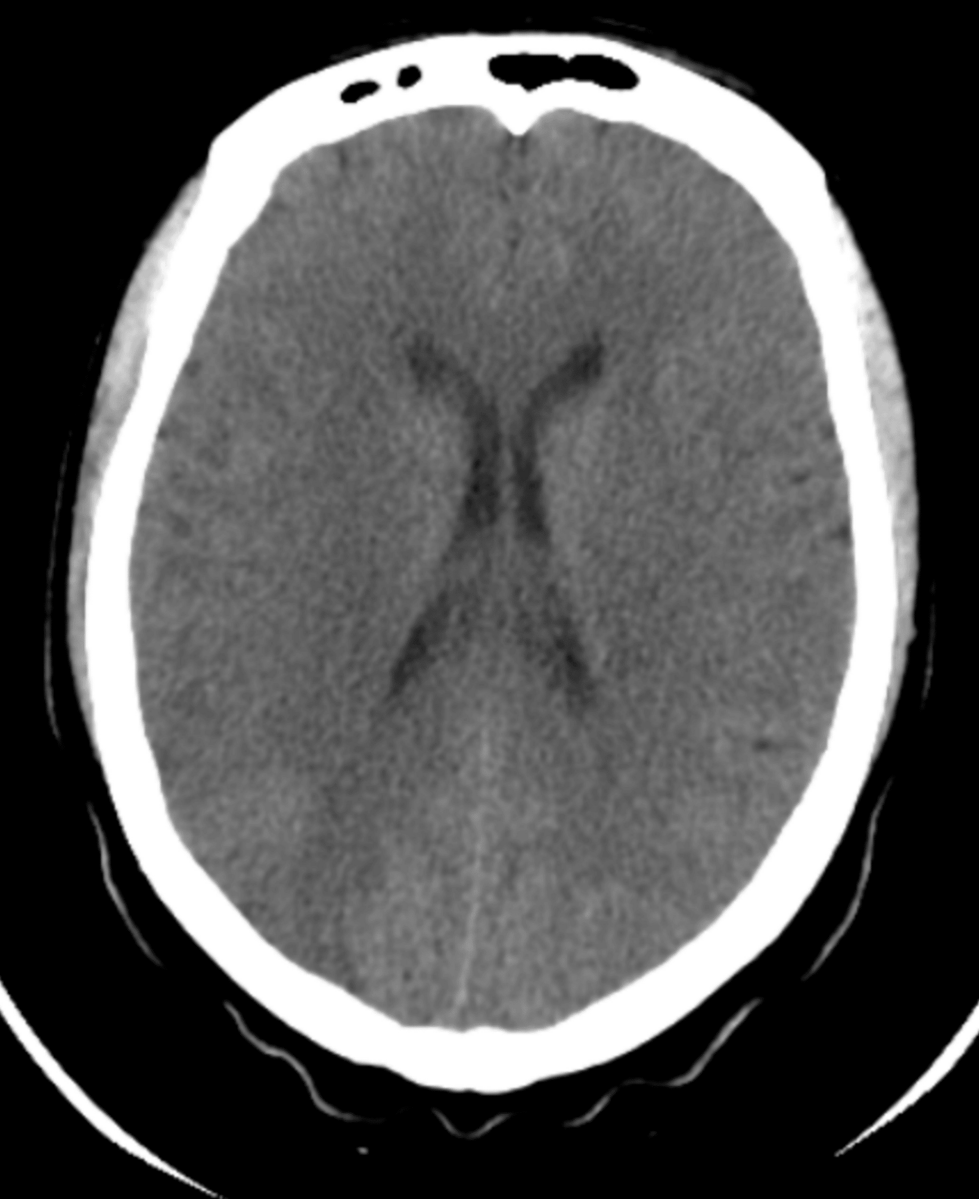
Initial non-contrast CT brain. Normal study.
